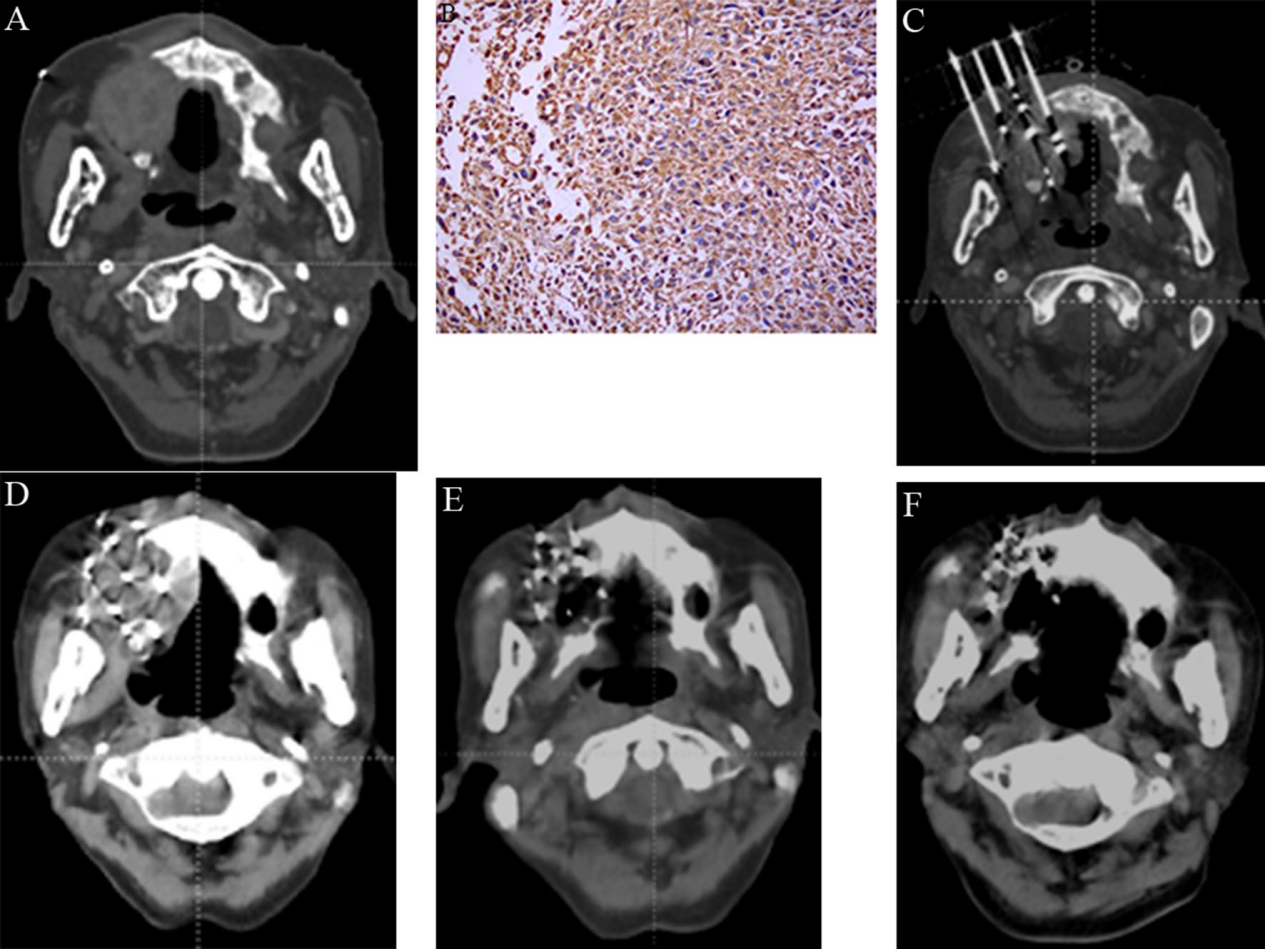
(A) Preoperative CT image of an 81-year-old male patient with maxillary sarcoma showed the tumor invaded the surrounding tissues. (B) Preoperative biopsy pathological pictures suggesting the undifferentiated sarcoma (or malignant fibrous histiocytoma). (C) In interventional surgery, the template-assisted CT-guided needle insertion image showed that the needle insertion angle and depth were good, and the large blood vessels were successfully avoided. (D): Re-examination at 3 days after intervention, CT showed uniform particle distribution in the lesion area. (E) CT image at 6 months after seed implantation showed that the lesion was significantly reduced, and a small amount of residual lesions could be observed on the edge. (F) At 1 year after intervention, CT image showed that the lesion disappeared completely, the boundary was clear, and the implanted particles gathered locally.
